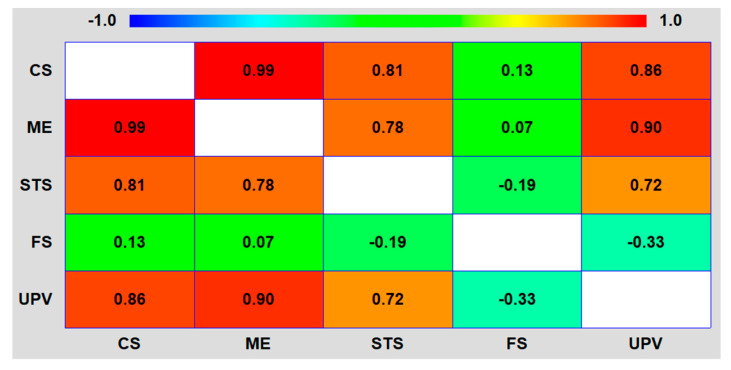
Pearson’s correlation matrix (legend: CS, compressive strength; ME, modulus of elasticity; STS, splitting tensile strength; FS, flexural strength; UPV, ultrasonic pulse velocity).
